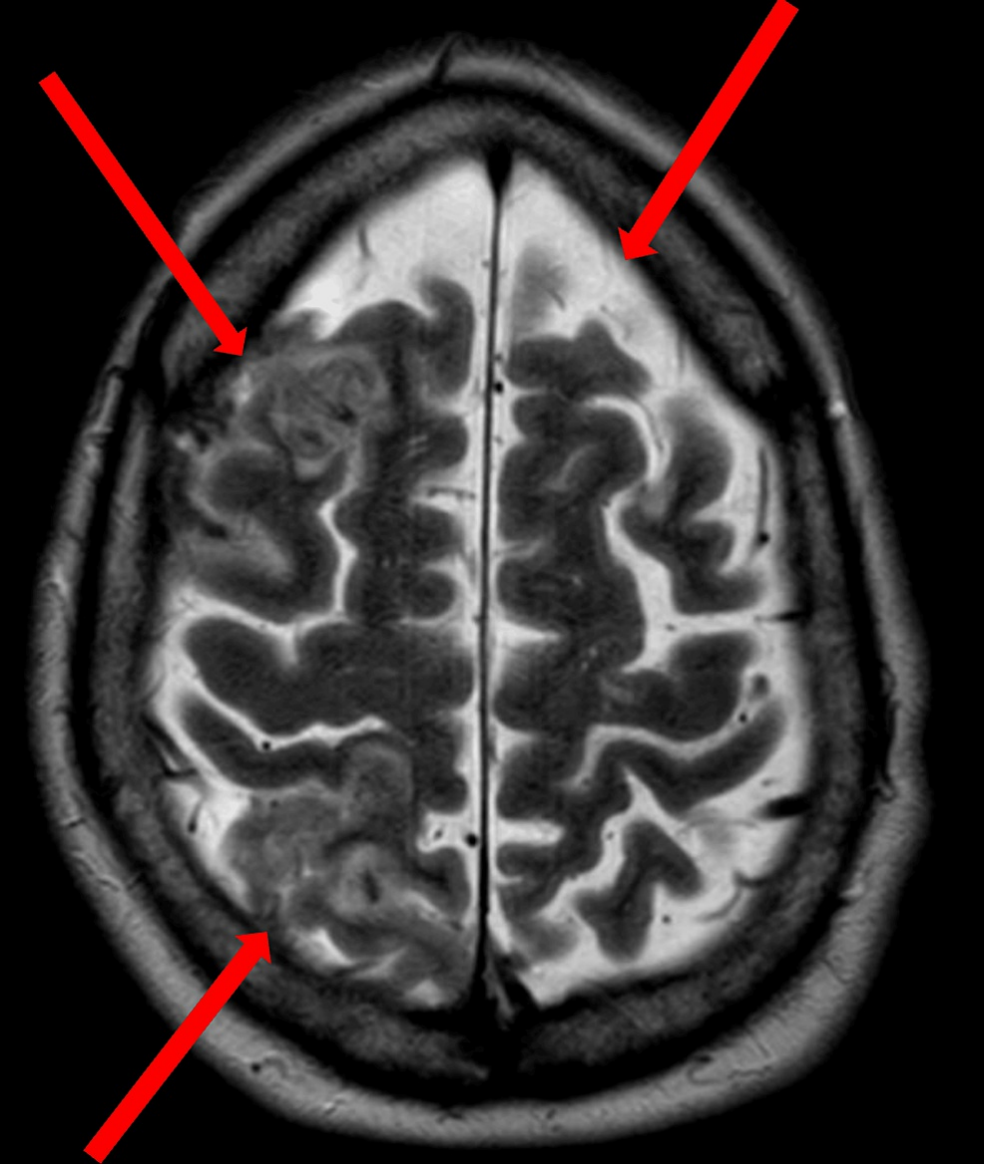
A T2-weighted MRI of the brain without contrast shows areas of increased signal, representing areas of acute subarachnoid blood and small cortical infarcts (arrows).
